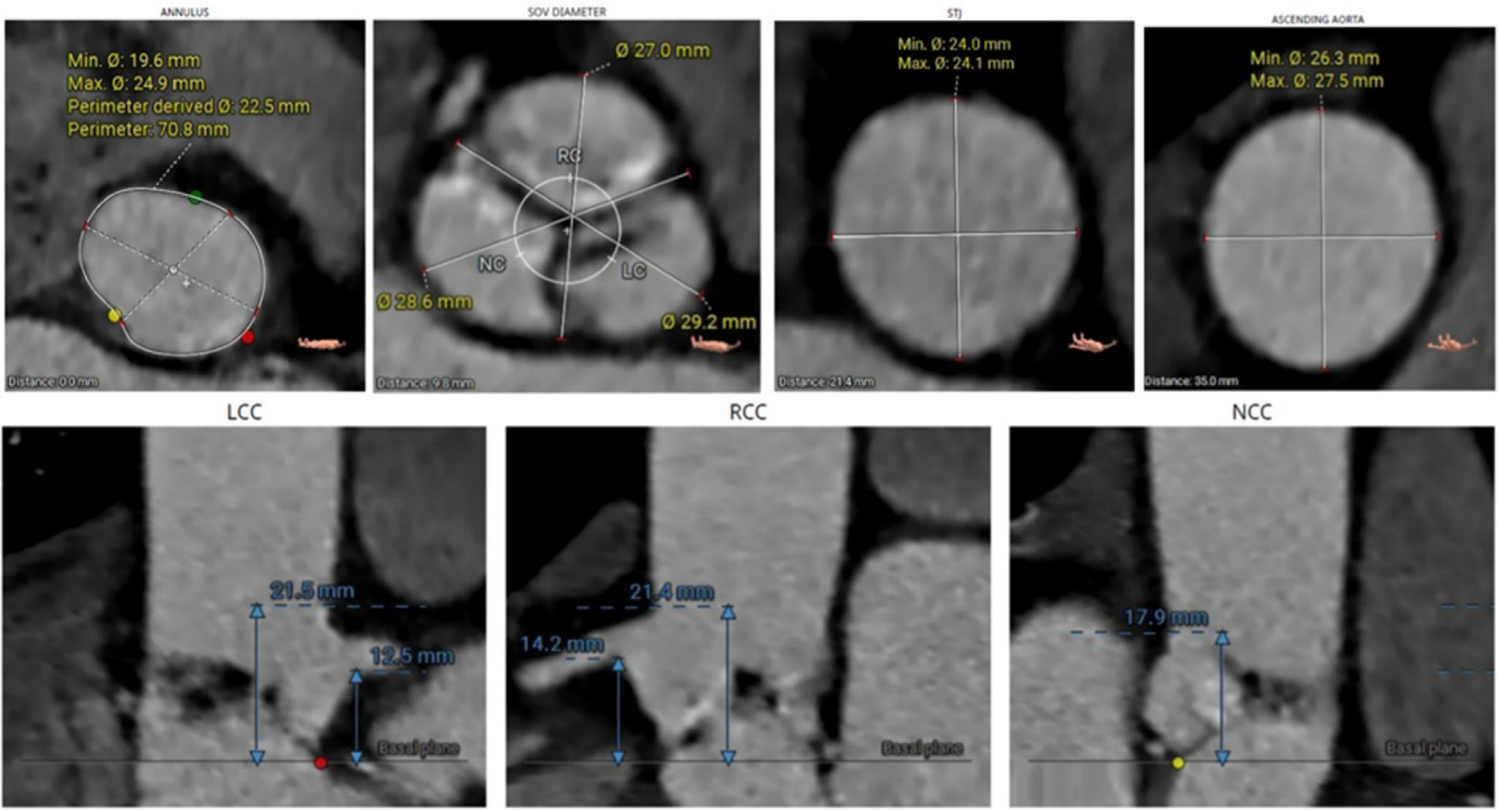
CT Measurements Obtained Prior to TAVR Computed tomography (CT) measurements obtained prior to transcatheter aortic valve replacement (TAVR) used for valve sizing. Note larger diameters of aorta compared to annulus. LC = left coronary; LCC = left coronary cusp; NC = noncoronary; NCC = noncoronary cusp; RC = right coronary; RCC = right coronary cusp; SOV = sinus of Valsalva.
